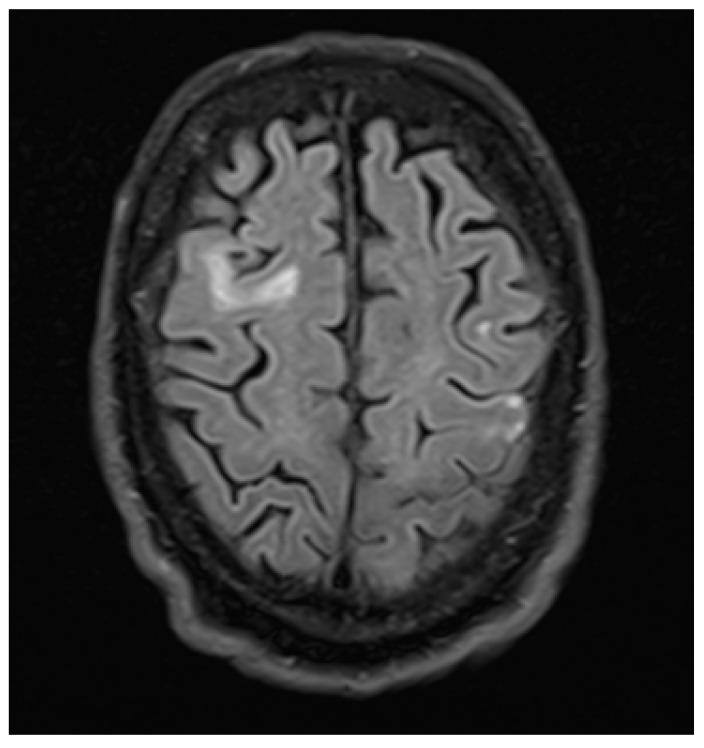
FLAIR cranial MRI image of the postoperative defect of the right FEF.
